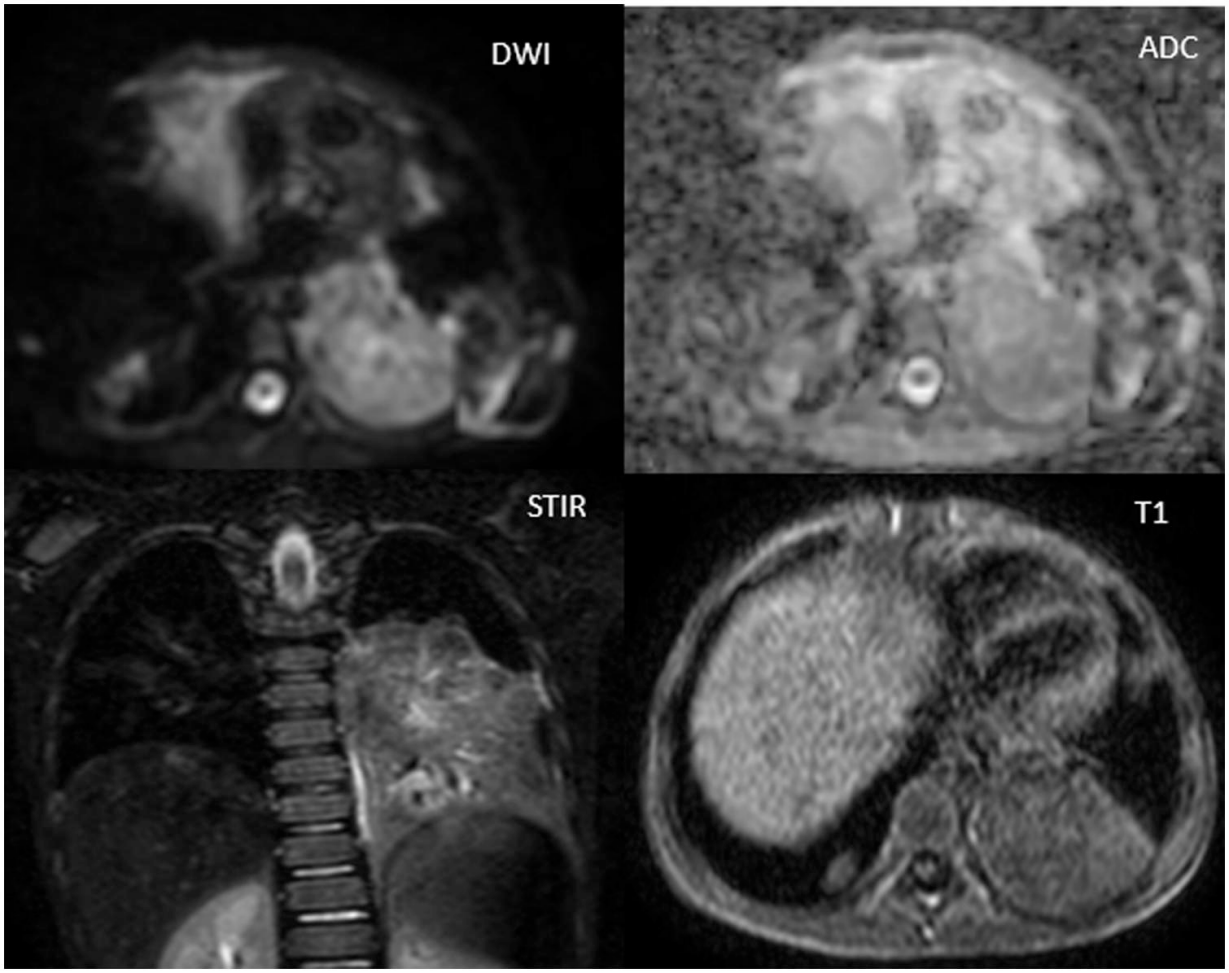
MRI thorax showing left parenchymal lesion appearing hypo-isointense on T1 and isointense on STIR images. No diffusion restriction in the mass.
